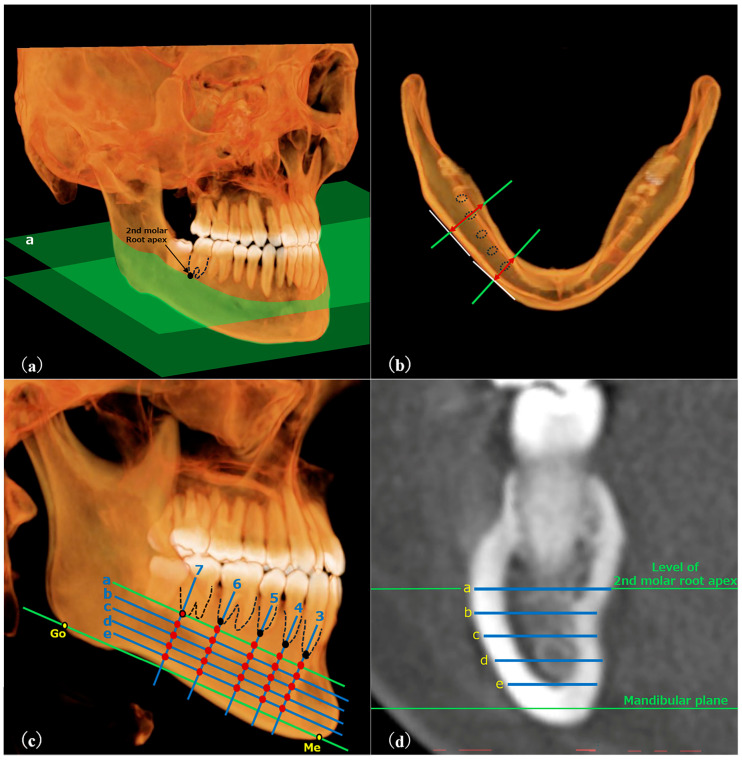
(a–d) CT images viewed with DICOM viewer. (a) Plane “a” was defined as that parallel to the mandibular plane and intersecting the distal root apex of the second molar. (b) A plane was established in plane “a” that passed through the root apex (distal apex for molars) and was perpendicular to the tangent of the mandibular buccal surface. (c) Five oblique lines were drawn perpendicular to the mandibular plane, intersecting the tooth apices. Four equidistant horizontal lines were drawn from plane “a” to the mandibular plane. Thickness was measured at 25 intersections. (d) An example of measurement at a certain cross-section.
